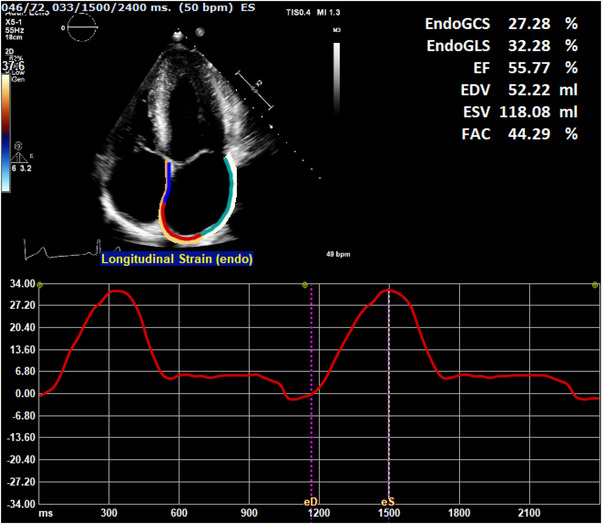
Example of left atrial reservoir strain measurement in a genotype‐positive, phenotype‐negative subject. Top left: Apical four‐chamber view with semi‐automatic overlay of left atrial wall. Bottom: Left atrial strain curve during two cardiac cycles. X‐axis: cardiac cycle in milliseconds, starting from end‐diastole. End‐systole marked during second cycle at 1500 ms. Y‐axis: Strain value (%) relative to the zero strain reference sat at end‐diastole. Top right: data extracted from left atrial wall tracing, *EndoGLS* represents relevant reservoir strain value, corresponding to the difference between peak strain at end‐systole and zero strain at end‐diastole.
